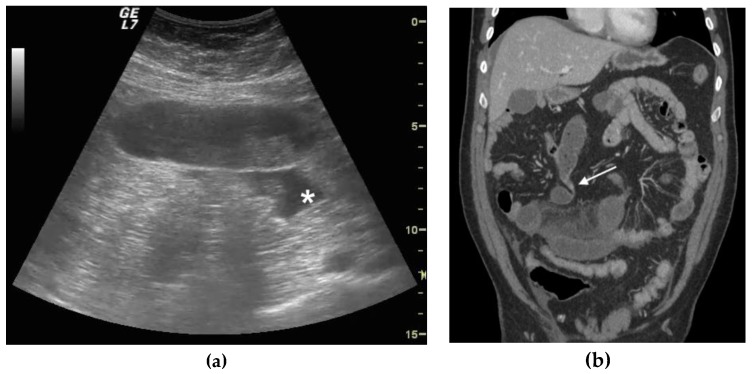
A 56-year-old male patient with virgin abdomen with compensated ileus. (a) Ultrasound long axis evaluation of a fluid-filled dilated small bowel loop, parietal was not thickened, free fluid was detected (*), peristalsis was ineffective with “back and forth” movements and defined decreased. (b) CT examination with IV contrast and coronal reconstruction. Small bowel fluid filled dilated loops were detected, and meso stranding and free fluid between bowel loops were also present. A focal point of stricture was evident (white arrow). At surgery, after release of adhesion, the bowel loop pinks up, peristalsis recovered and resection was avoided.
